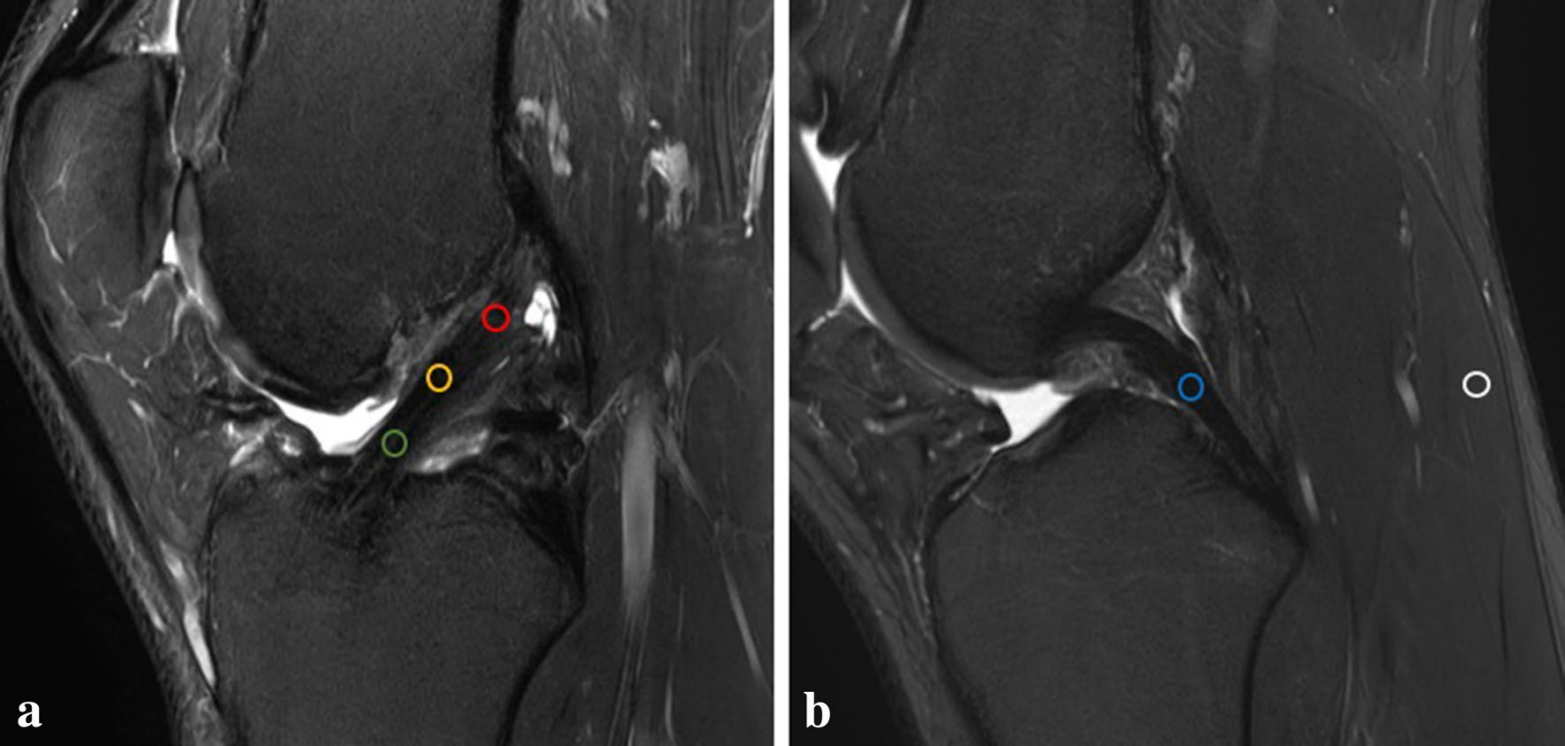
Magnetic resonance imaging signal intensity was measured within a standardized 4 mm diameter circle at the respective region of interest at (A) the proximal (red), mid-substance (yellow) and distal (green) portion of the anterior cruciate ligament autograft and (B) at the mid-substance of the posterior cruciate ligament (blue) and the medial head of the gastrocnemius (white)
